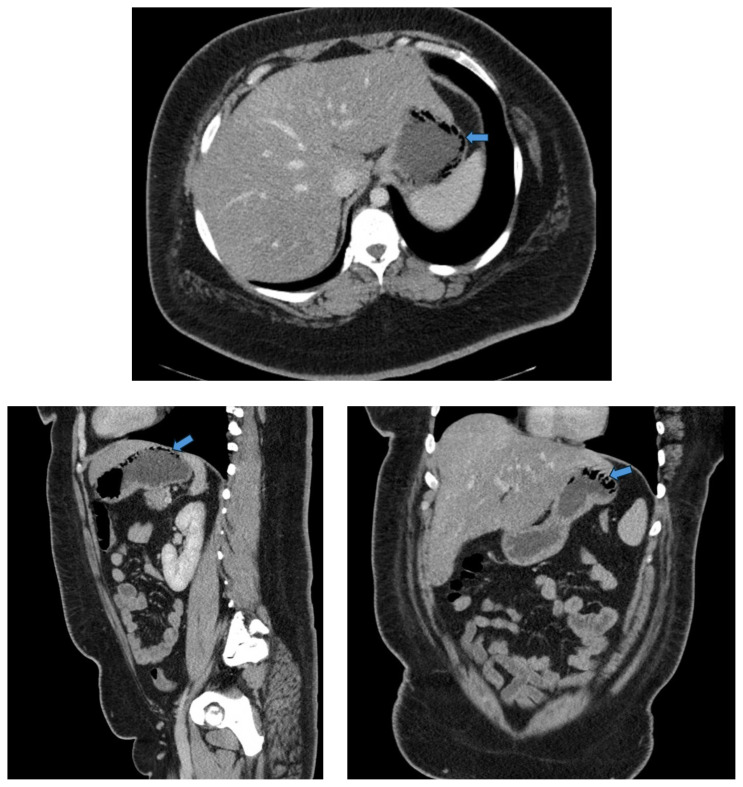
CT Video Link: https://youtu.be/zMVLLRbTB0w
